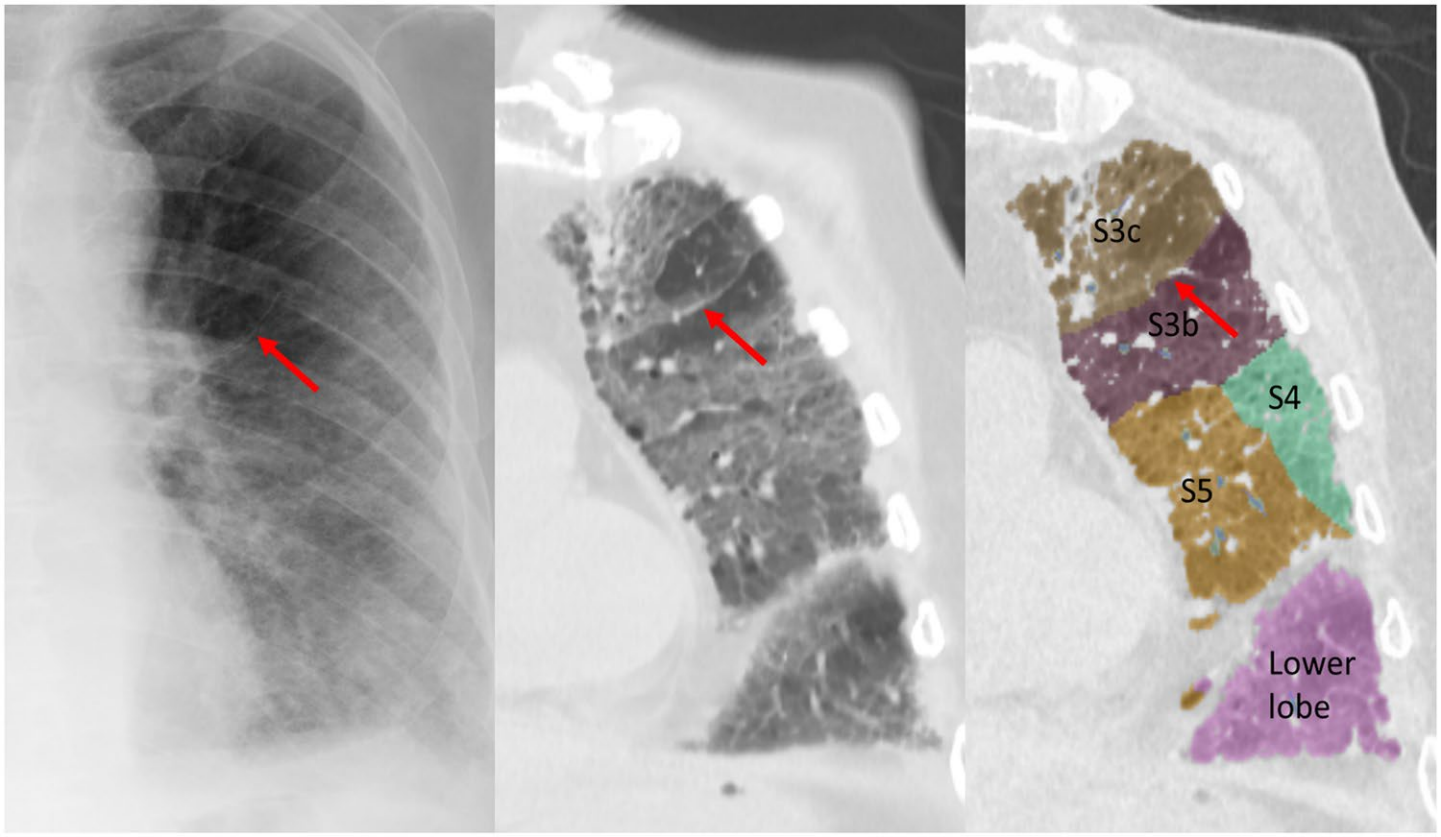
Case 6. Line 9. 88-year-old man with prostate cancer. Chest X-ray shows the Kerley A-line in the left lung. The line corresponds to the thickened septal line on CT and matched with the segmented lines between S3b and S3c by 3D segmentation analysis
